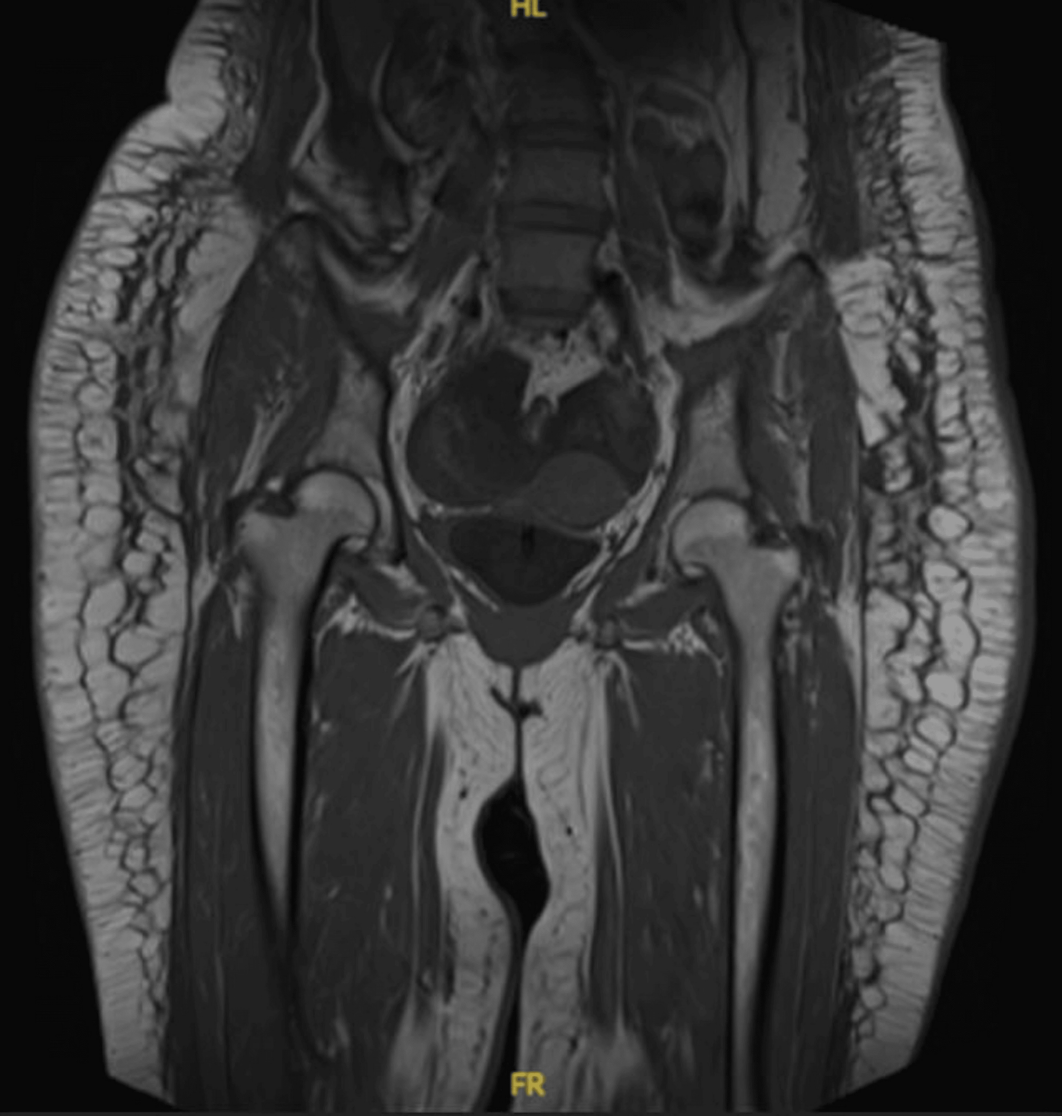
MRI of the bilateral thighs Bilateral extensive subcutaneous and muscle edema particularly affecting the proximal muscles MRI: magnetic resonance imaging
